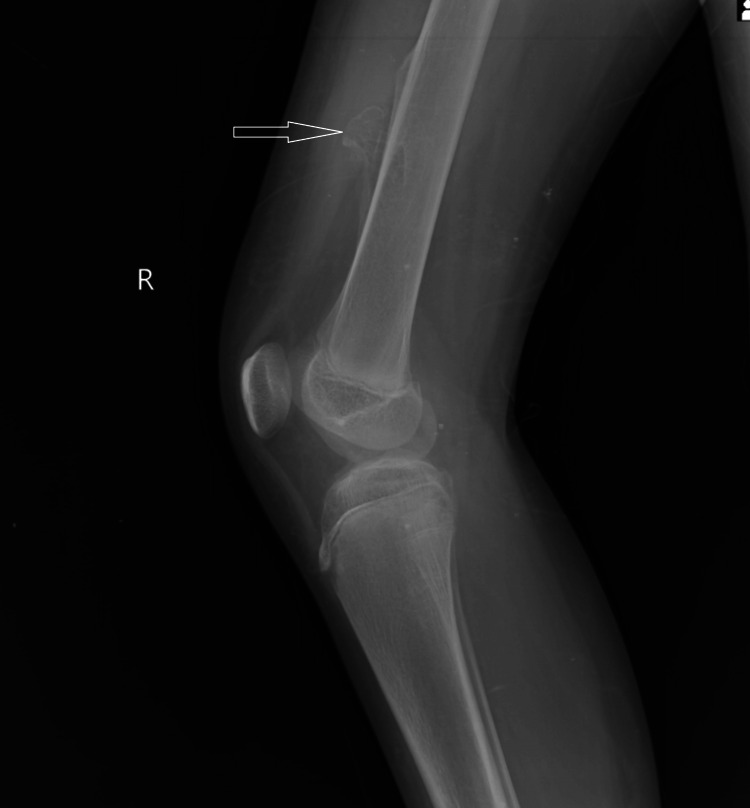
The lateral view radiograph of the right knee with the distal femur and the proximal tibia in an immature skeleton shows a sessile bony outgrowth in the meta-diaphyseal location of the distal femur.
